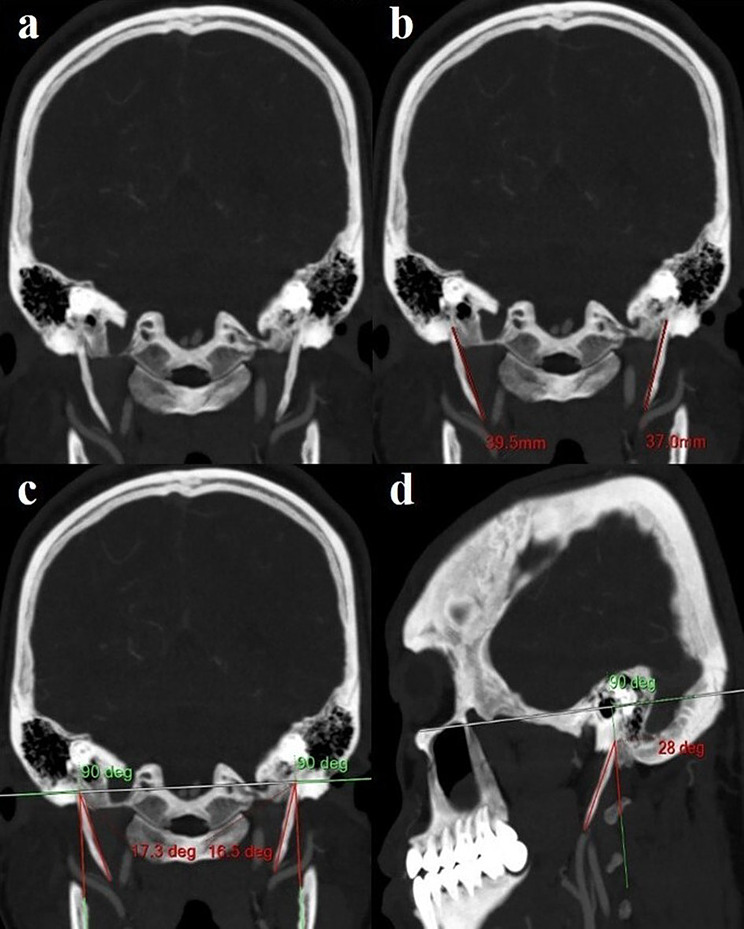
CTA-based measurements of the SP. a Coronal oblique MPR image showing complete visualization of both SP. b The red line represents the SP length, measured from the midpoint of the SP base to the tip. c The white line is the horizontal baseline, the green line is the vertical reference axis, and the red angle represents the medial inclination angle. d The white line is the OML used as the horizontal baseline, the green line is the perpendicular reference axis, and the red angle represents the anterior inclination angle
